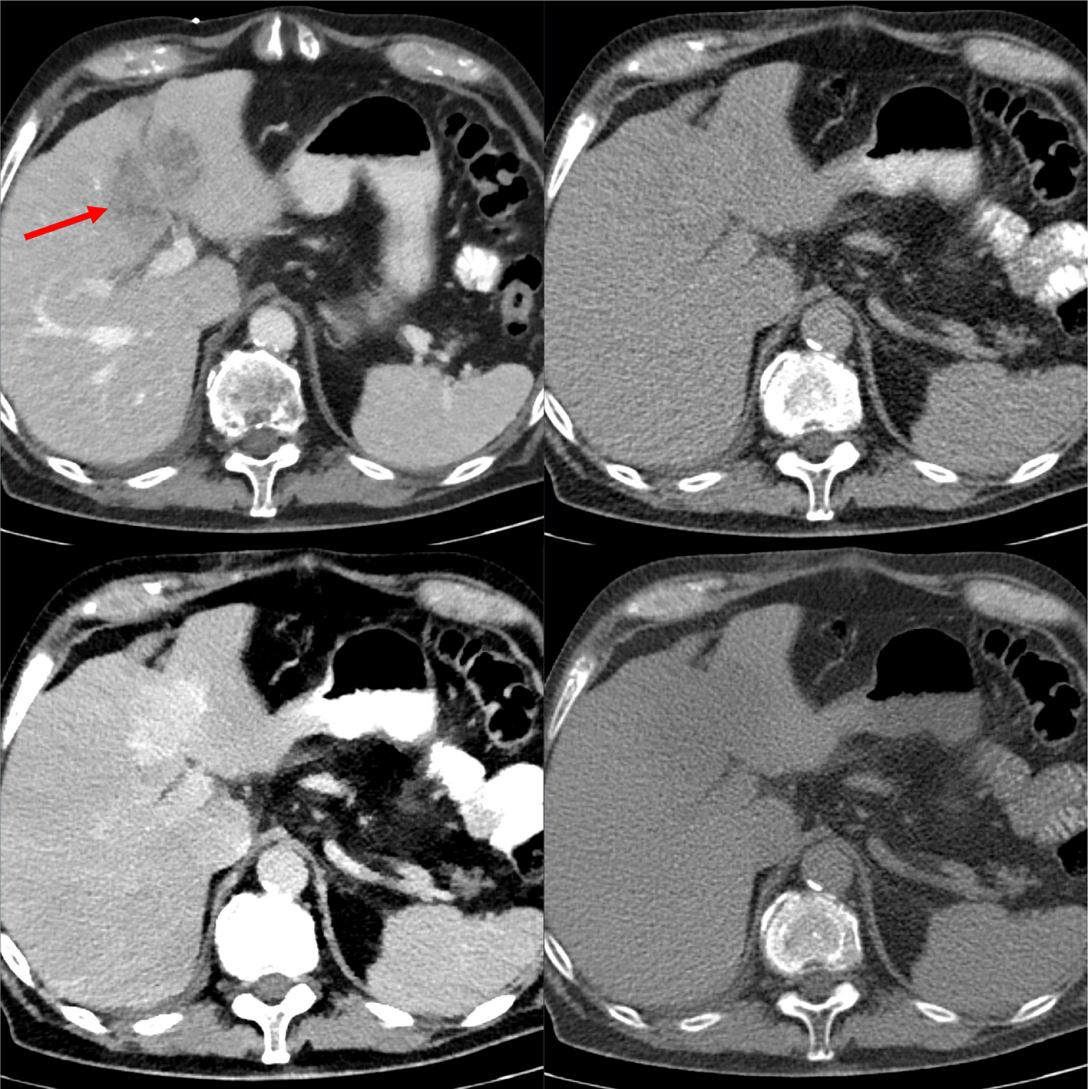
Patient #20 with a primary intrahepatic cholangiocarcinoma. Shown are the diagnostic CT in the venous phase (top left), as well as the corresponding biopsy images. The lesion is best visible in VMI-40 image (bottom left) due to hyperdensity but also well visible in the VNC image (bottom right) due to subtraction of iodine (hypodensity). The conventional reconstruction (top right) shows the worst visibility
